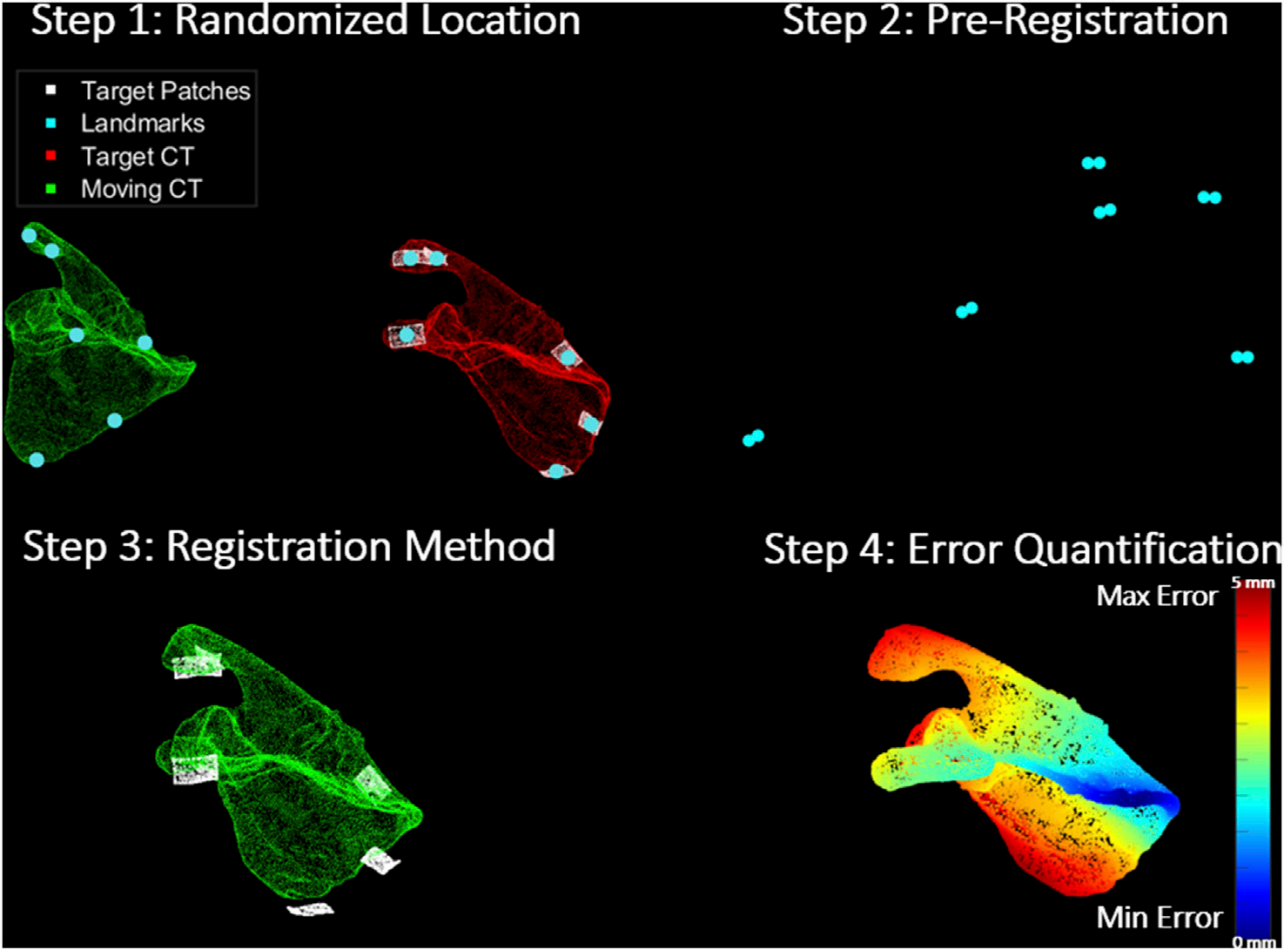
Registration Protocol: Step 1 shows the moving CT (green) transformed to an arbitrary location away from their true pose, target CT (red) and its corresponding target patches (white). It also shows the corresponding landmarks (turquoise) on the surface of each set. Step 2 depicts the pre-registration approach (coarse registration) utilized to obtain a positional initial estimate of the moving CT with respect to the target patches through the alignment of the corresponding landmarks from both sets. In step 3, the registration methods (ICP and CPD) are applied to register the moving CT to the target patches. Finally, step 4 shows the error quantification procedure which calculates the RMSE between the target and estimated moving CT pose in a point-by-point manner. The scapula in the figure is colour-coded based on how far each point in the moving CT of the scapula is from its corresponding true pose in the target CT.
